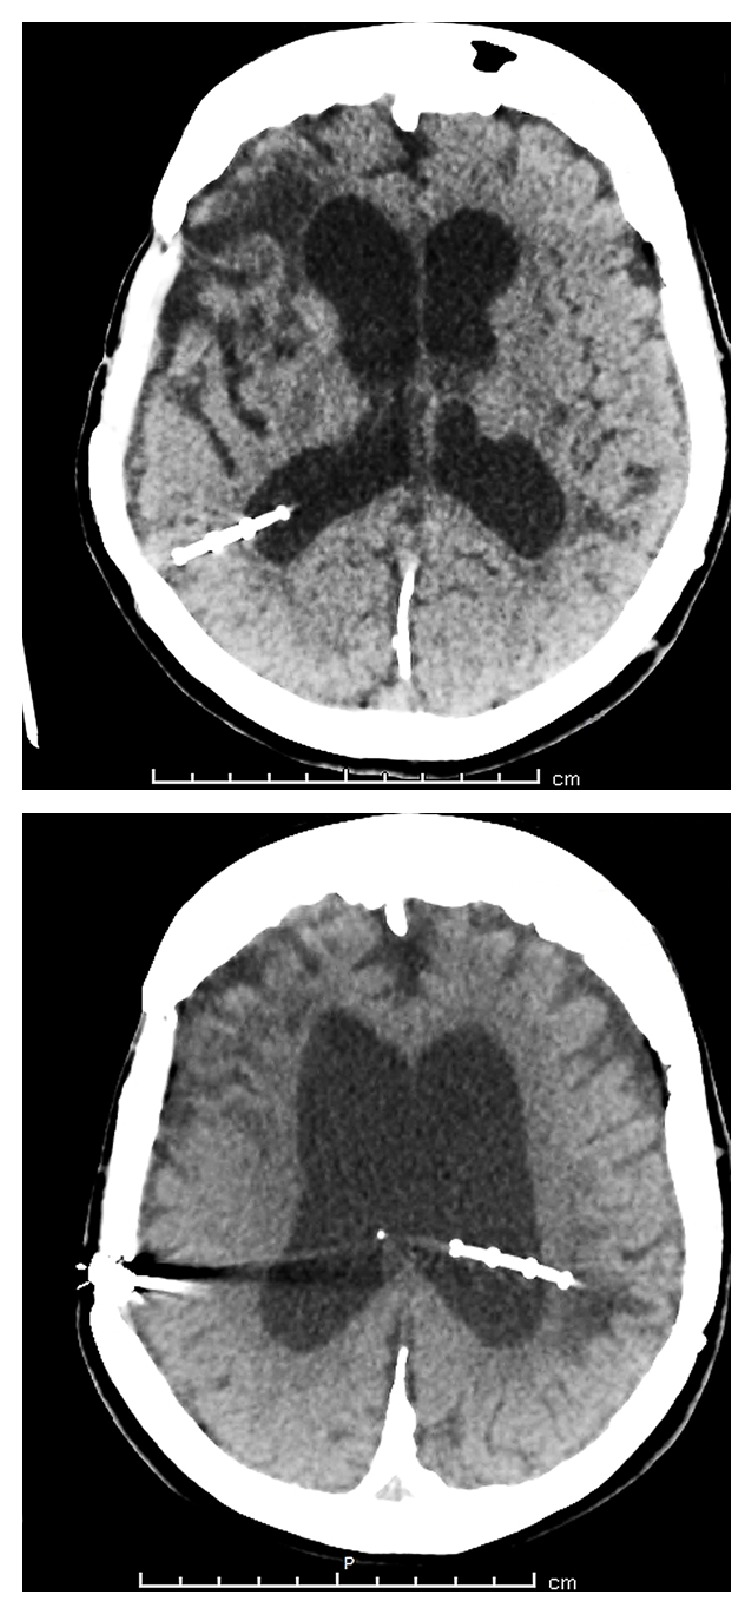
CT-brain scan demonstrates no intracranial free air and VP shunt in place in patient's 2 months prior presentation.
